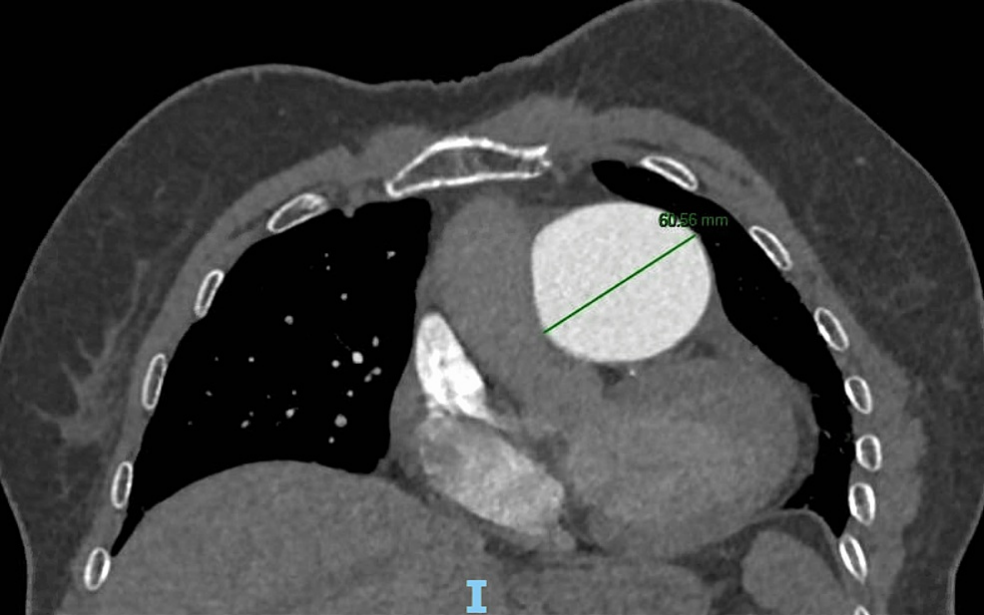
Maximum diameter of PAA on axial plane of pre-operative CT scan PAA: Pulmonary artery aneurysm
